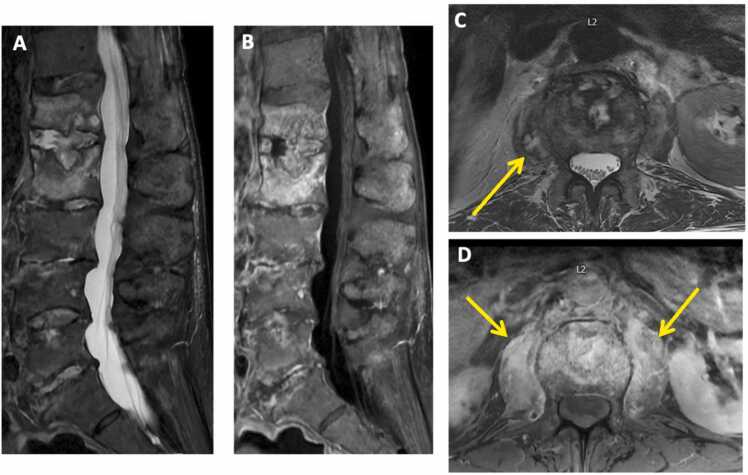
MRI confirms discitis-osteomyelitis changes observed on CT. Sagittal STIR (A) and post-contrast images (B) demonstrate prominent edema and enhancement of the L1 and L2 vertebral bodies and within the L1-L2 intervertebral disc, compatible with discitis-osteomyelitis. There is also mild edema and enhancement of the L3-L4 vertebral endplates, which in conjunction with new erosive endplate changes seen on the CT, are suggestive of early discitis-osteomyelitis at this level. C. Axial STIR image demonstrates a small heterogeneous collection in the right psoas muscle. D. There is no definite rim-enhancement on the Axial T1 post-contrast image. Therefore, this is most compatible with a phlegmon, rather than a frank abscess. Axial T1 post-contrast image also demonstrates diffuse enhancement of the bilateral psoas muscles compatible with inflammatory change.
